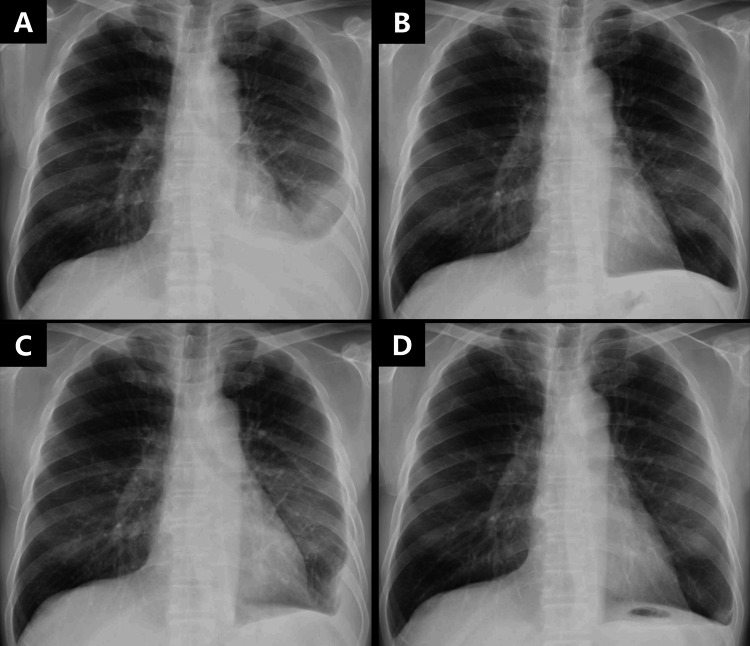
Changes in rheumatoid pleural effusion (RPE) observed via chest X-ray. (A) Left-sided RPE at initial presentation. (B) Improvement in RPE at week 7 after prednisolone initiation. (C) Relapse of RPE at week 35 after prednisolone tapering. (D) Decrease in RPE at week 6 after sarilumab initiation.
